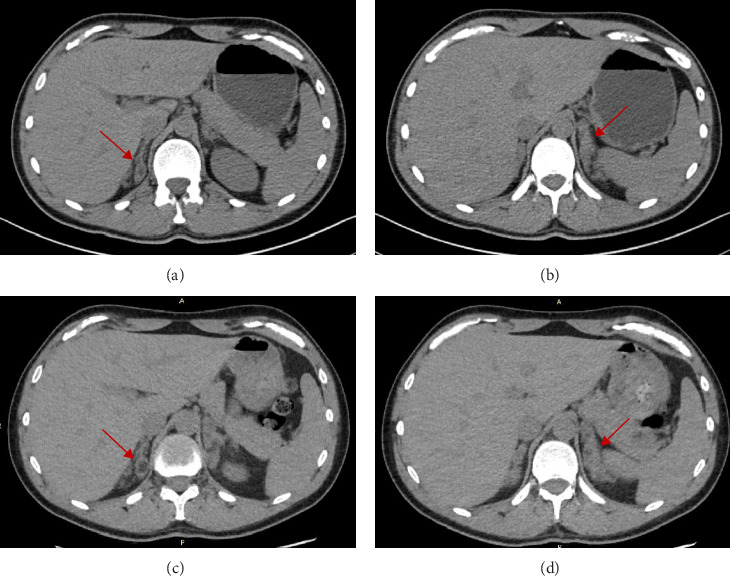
The CT scan at DR-HC start (A and B panels) and at last evaluation, 4 years later (C and D panels). A and C panels highlighted right adrenal enlargement with a hypodense nodule of the medial limb of 13 mm × 18 mm that remained stable during DR-HC treatment; B and D panels showed, instead, a diffuse enlargement of the left adrenal gland due to a long-term uncontrolled disease.
